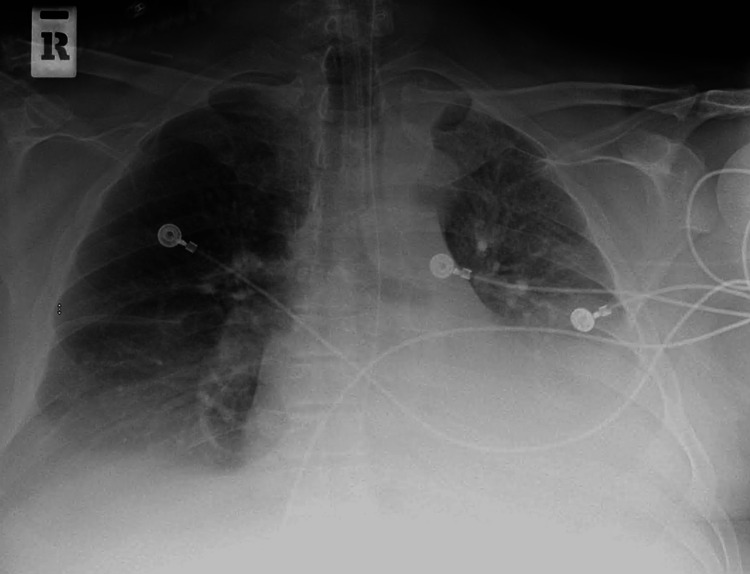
X-ray chest showing bilateral (left greater than right) interstitial and bibasilar infiltrates with pleural effusion
